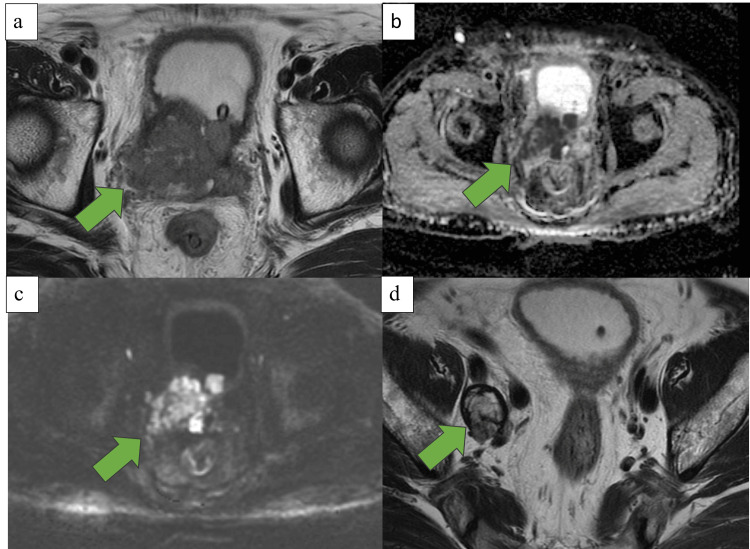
Magnetic resonance imaging a, b, c: T2WI and apparent diffusion coefficient and diffusion-weighted imaging showing prostatic enlargement with a mixed signal mass of 6.0 cm × 5.2 cm × 6.4 cm and prostate cancer with bladder invasion. d: T2WI showing right obturator lymph node enlargement with a mixed signal mass of 2.5 cm × 3.0 cm × 2.7 cm. T2WI, T2-weighted imaging.
